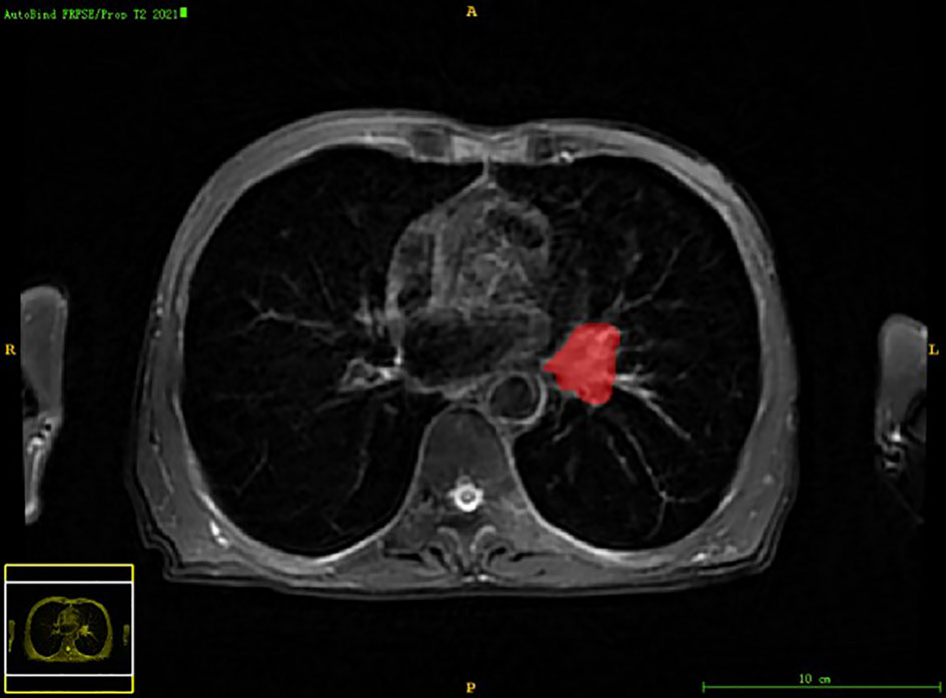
The lesion ROIs of axial MRI sequence.
